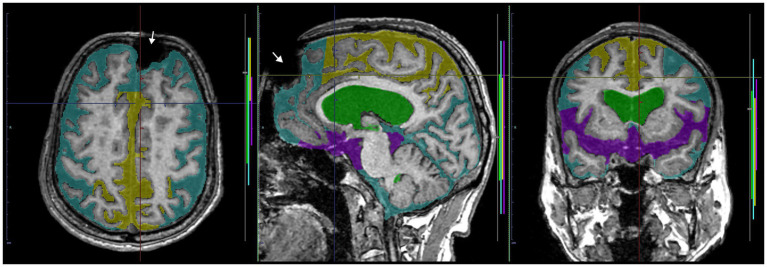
A case of discrepancy in DESH determination between AI and expert. MRI of an 84-year-old male volunteer, who claimed no specific history of head trauma showed a signal deficit (white arrow) in the left frontal region due to a metal artifact. The AI automatically judged the presence of DESH (softmax probability score: 1.0), VD (1.0), SFD (0.75), and the absence of THC (0.84), while the expert judged the presence of VD but not DESH, THC, or SFD. This case should have been excluded from the study.
